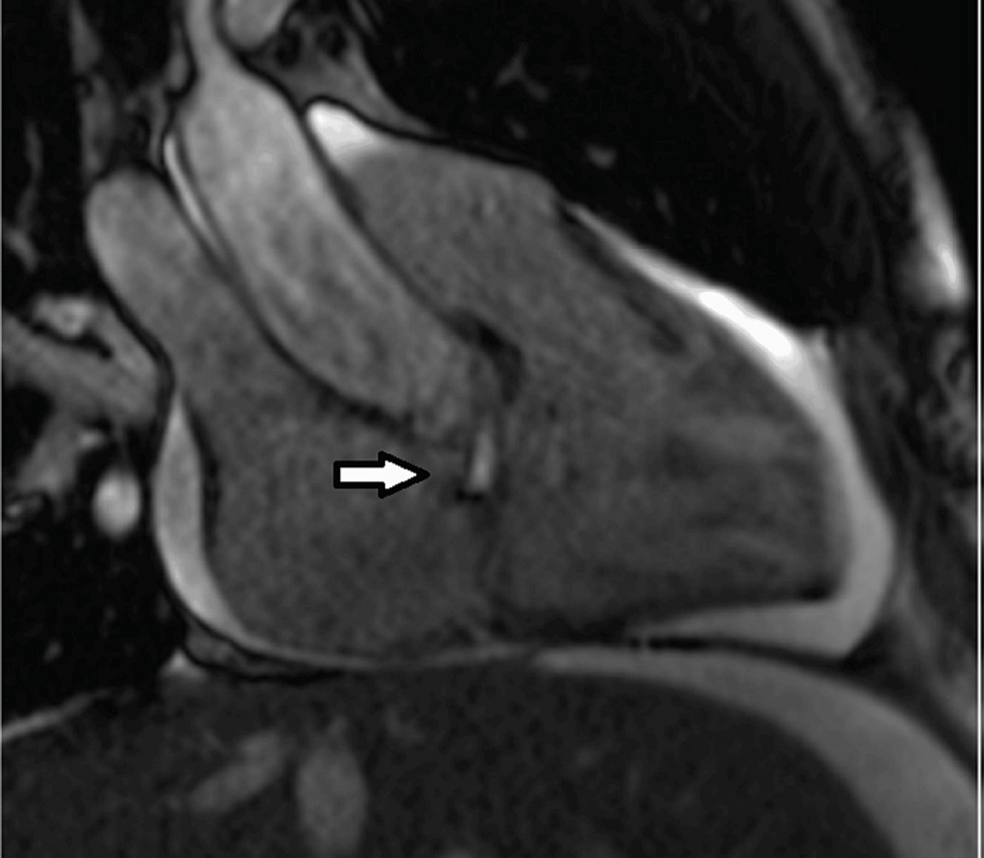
Cardiac Magnetic Resonance Imaging (MRI). Still frame of a modified right ventricular inflow-outflow steady-state free precession (SSFP) cine MRI, including the aortic root and showing a SOVAR into the right atrium just above the plane of the tricuspid valve (arrow shows the jet entering the right atrium).
